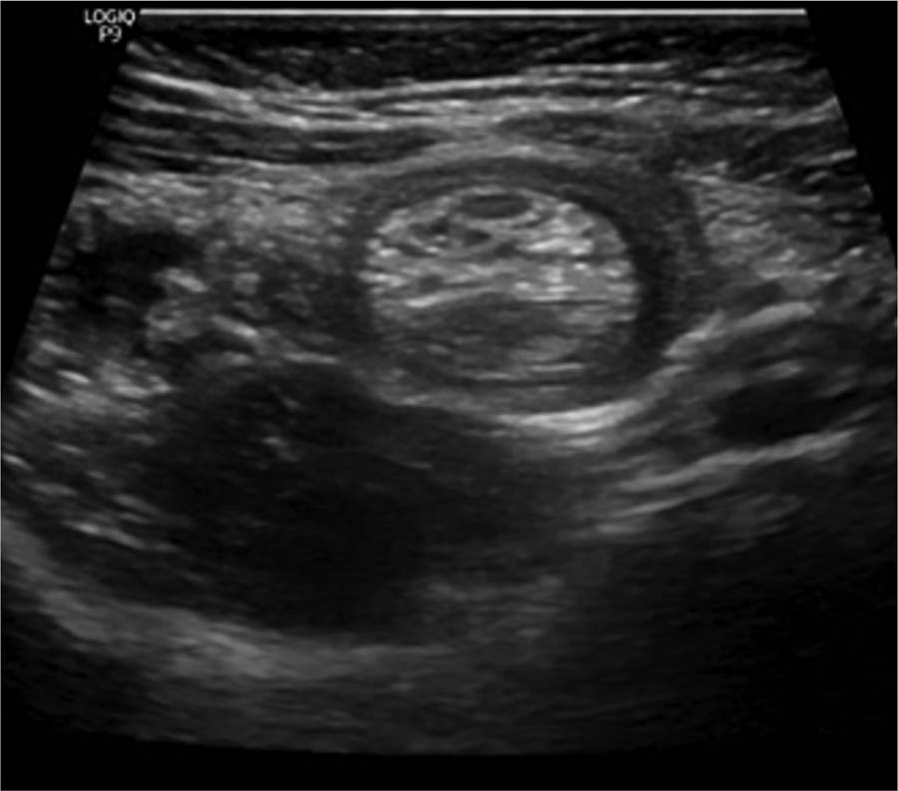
Ultrasound of the abdomen showed a target sign of intussusception (5th attack).
